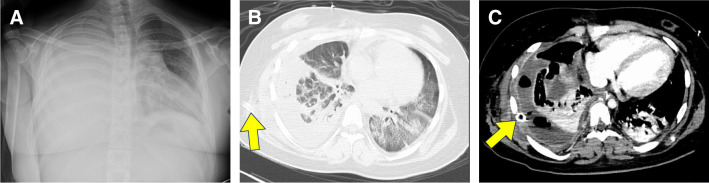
Preoperative findings. The drainage tube is shown as a yellow arrow. (A) Chest radiograph at the referring hospital shows pleural effusion occupying the entire right lung with deviation of the trachea and mediastinum. (B) CT after right chest drainage reveals infiltrative shadows in both lung fields, leading to a diagnosis of parapneumonic pleural effusion. (C) CT on the eighth day of hospitalization reveals thickening of the visceral pleura and multiloculated abscess cavities, leading to the diagnosis of acute empyema.
